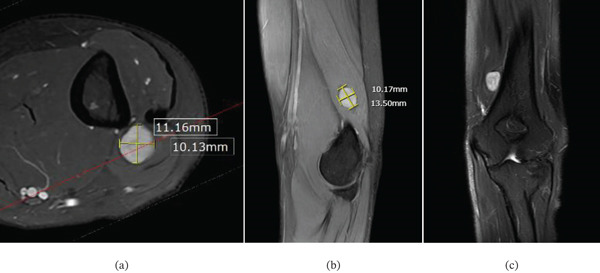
(a) T2 axial cut MRI image depicting the hyperintense tumour located within the medial head of the triceps brachii measuring 10 mm in the anteroposterior direction and 11 mm transversely. (b) A fat saturated sagittal MRI image depicting the hyperintense lesion measuring 13.5 mm craniocaudally. (c) A fat saturated coronal MRI image following contrast demonstrating tumour enhancement.
